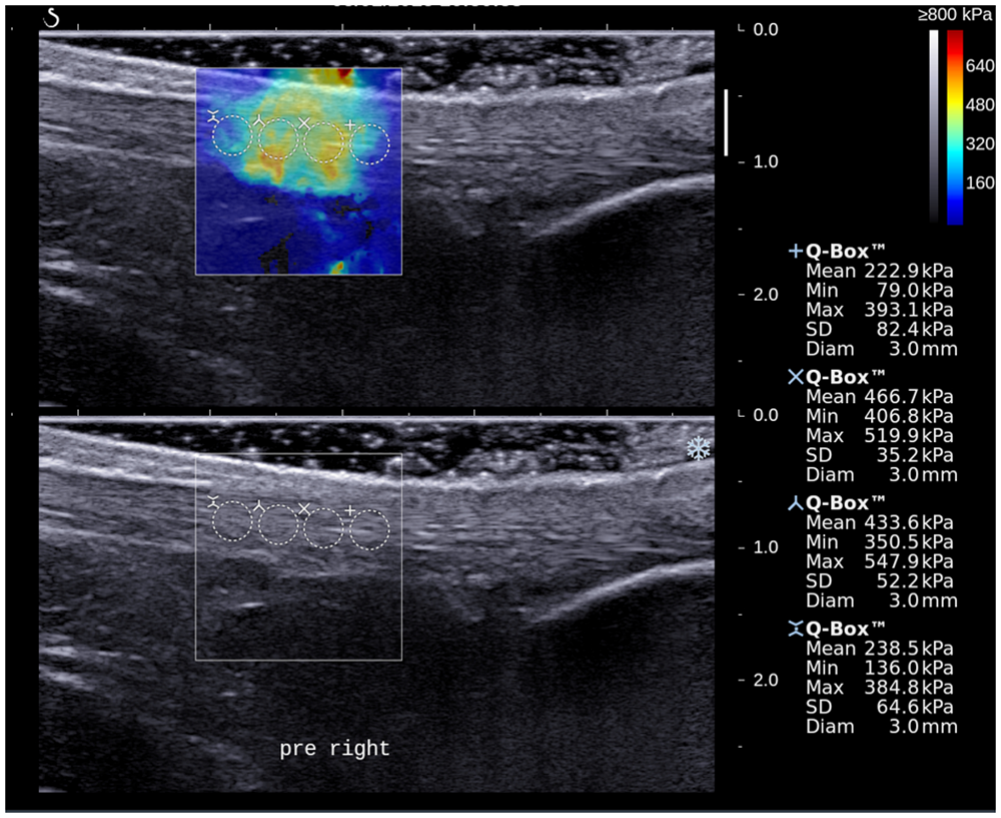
A longitudinal shear wave elastogram (upper) and the corresponding grey scale sonogram (lower) show an Achilles tendon on the non-dominant leg of a subject before stretching exercise. Four Q-boxes with a diameter of 3 mm were placed along the long axis of tendon for the measurement of tendon stiffness. Different stiffness parameters are shown on the right side of the image.
